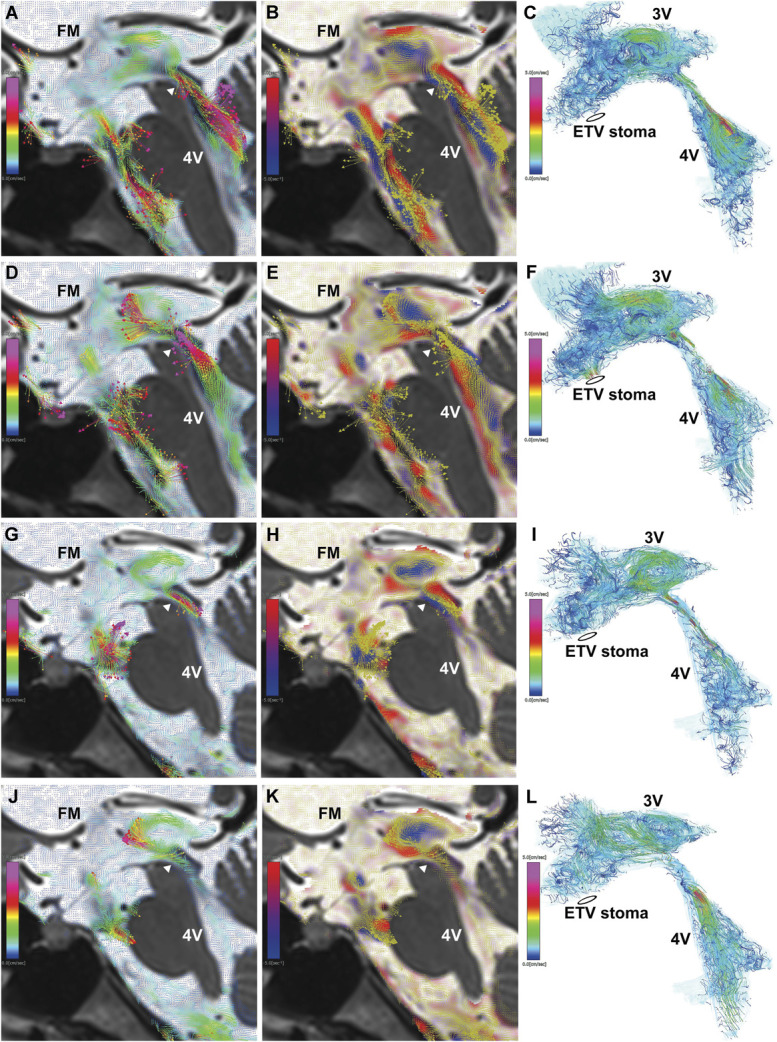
Communicating hydrocephalus (case 3). A-F, 4D flow MRI after ETV and before VPS. G-L, 4D flow MRI after VPS. A, D, and G, Velocity maps of 2D sagittal views, with colored arrows indicating velocity vectors. B, E, and H, Vorticity maps of 2D sagittal views, showing clockwise vortices in blue, counterclockwise vortices in red, and overlaid yellow velocity vectors. C, F, and I, Streamlines of CSF motion during a heartbeat in the 3D view; white arrowhead, upper end of the cerebral aqueduct. Rapid CSF flow from the cerebral aqueduct into the 4V and reversed rapid CSF flow from the cerebral aqueduct into the 3V were observed. A clockwise vortex formed in the posterior 3V behind the interthalamic adhesion (Massa intermedia). The fenestration in the 3V floor (ETV stoma) exhibited rapid, repetitive bidirectional flow within a single cardiac cycle. 2D, 2-dimensional; 3D, 3-dimensional; 3V, third ventricle; 4D, 4-dimensional; 4V, fourth ventricle; CSF, cerebrospinal fluid; ETV, endoscopic third ventriculostomy; FM, foramen of Monro; VPS, ventriculoperitoneal shunting.
